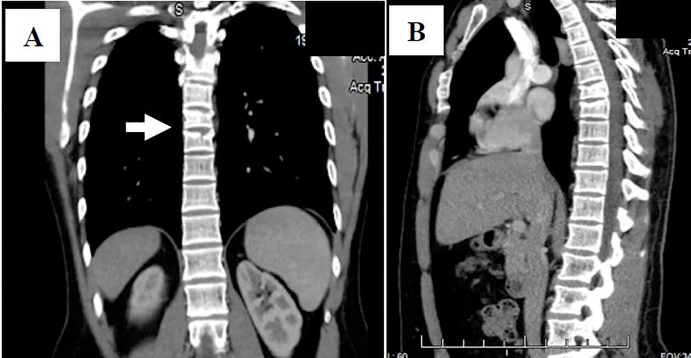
(A) Computed tomography of the chest (coronal view) showing fracture deformities of the thoracic 6th – 8th vertebral bodies (white arrow); (B) Sagittal view.
